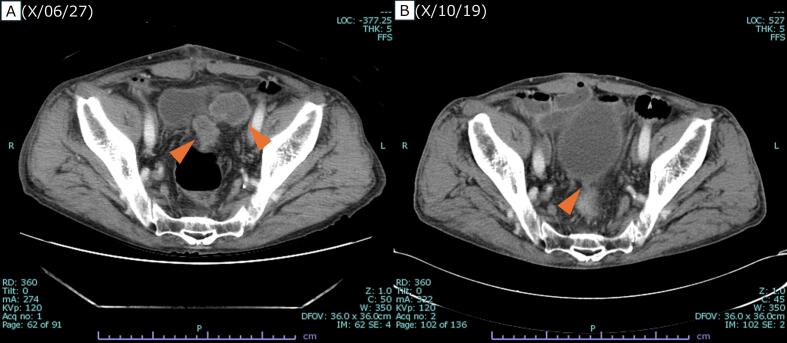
Contrast-enhanced CT after five courses of chemotherapy. Significant reduction in the size of the peritoneal nodules was observed following five cycles of mFOLFOX6 plus cetuximab. A, Before initiation of chemotherapy. B, After five courses of chemotherapy.
